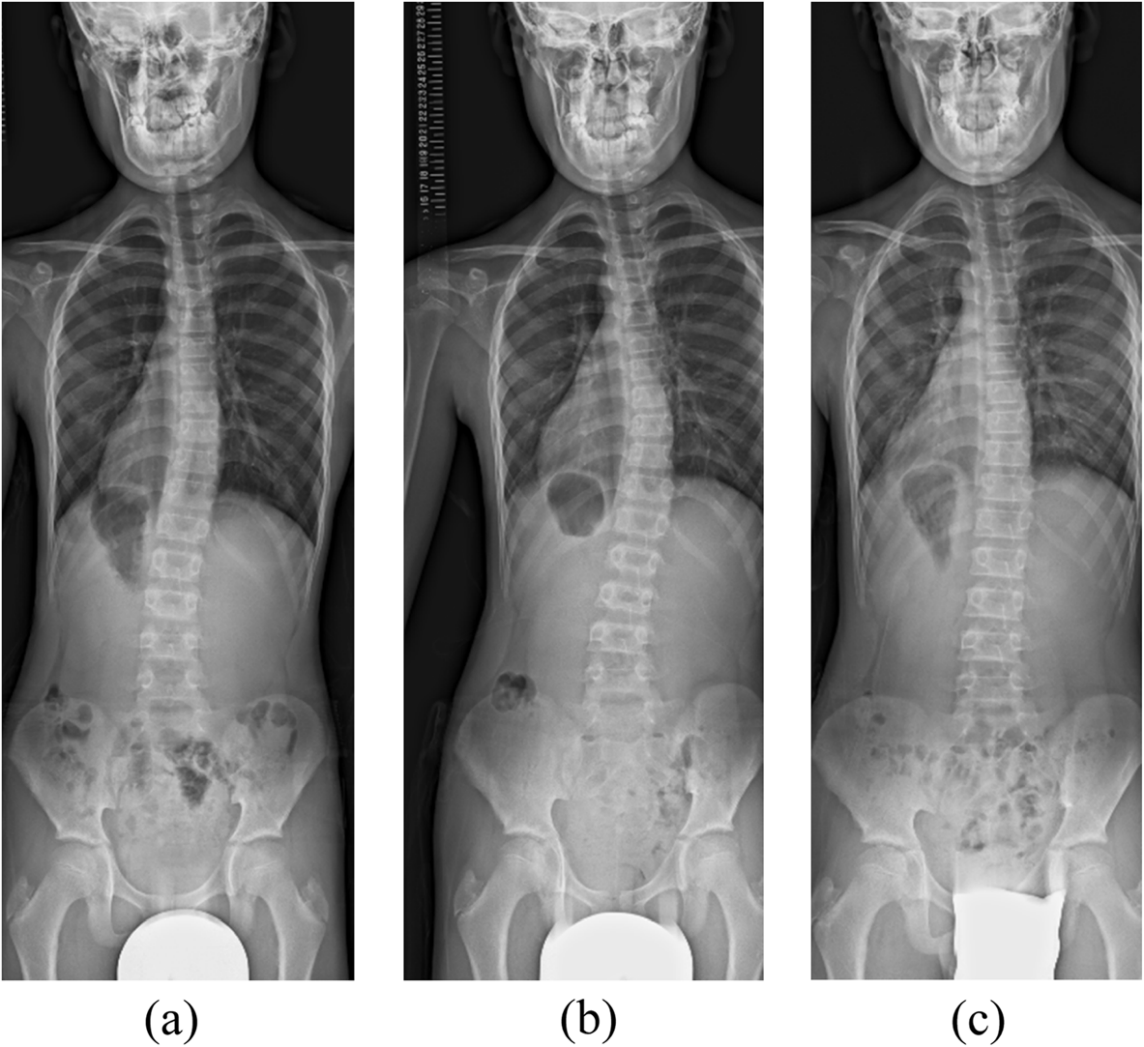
Radiographs during brace treatment. a At 3 months brace treatment, the scoliotic curve had improved to 16 degrees (T5-L2), although the curve had reversed into a right convex curve. b Scoliotic angle had increased to 21 degrees (T5-L2) at 7 months of brace treatment. c After another adjustment of the brace, his right convex scoliotic angle improved to 13 degrees (T4-L2) at 15 months of treatment
